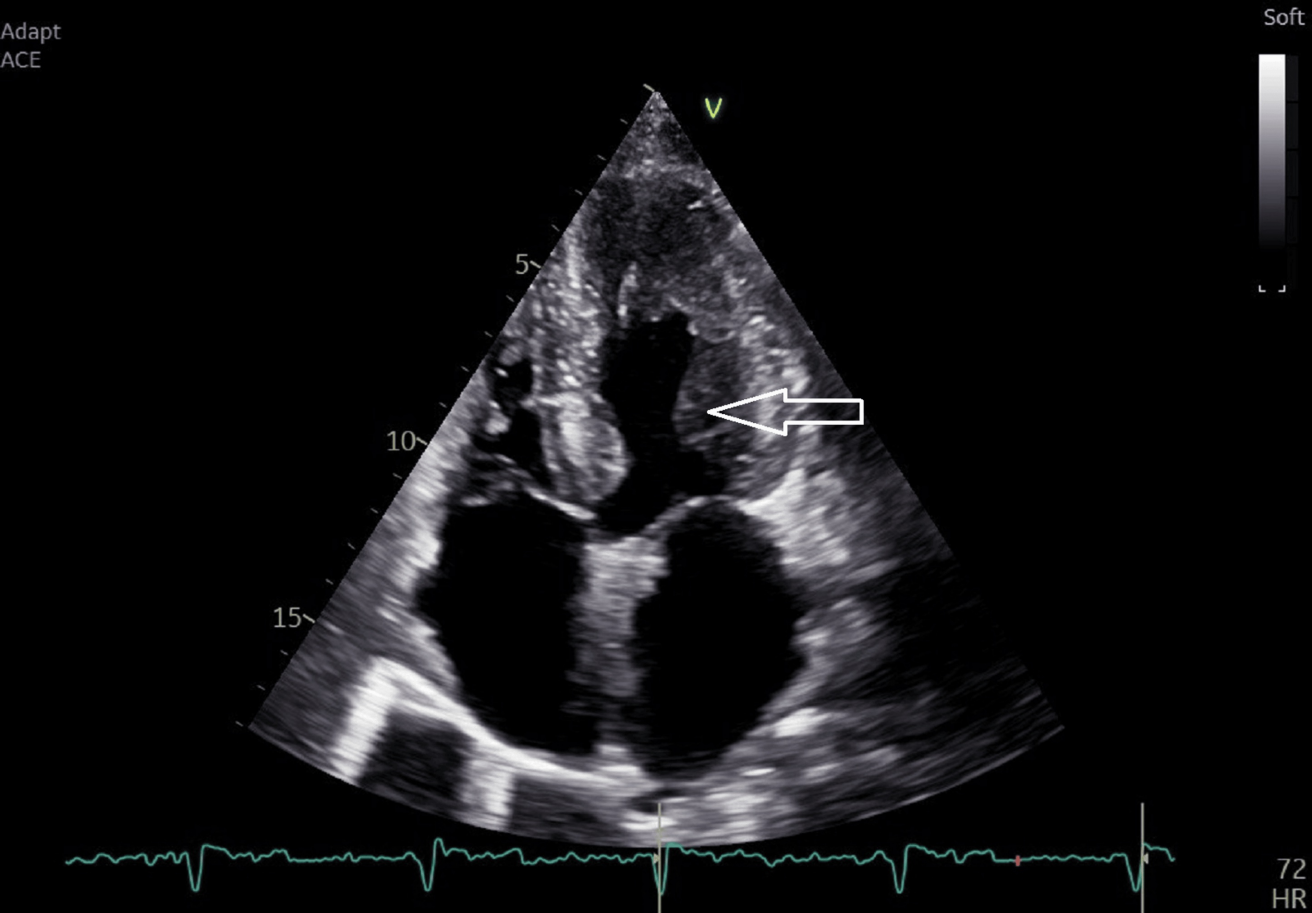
TTE showing the Ace-of-Spades configuration with papillary muscle hypertrophy TTE: trans-thoracic echocardiography
